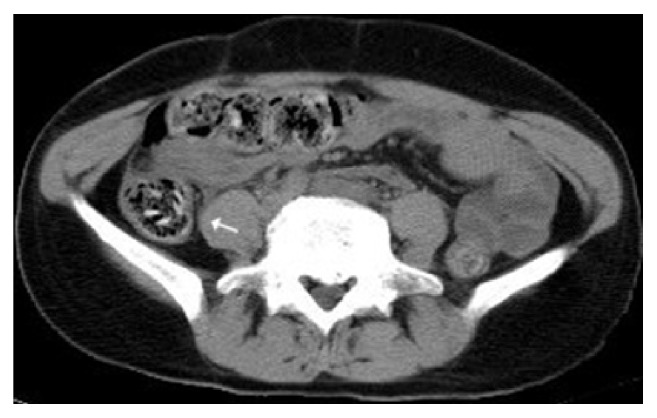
CT imaging; intraluminal phytobezoar appearance with persimmon seeds (arrow).
